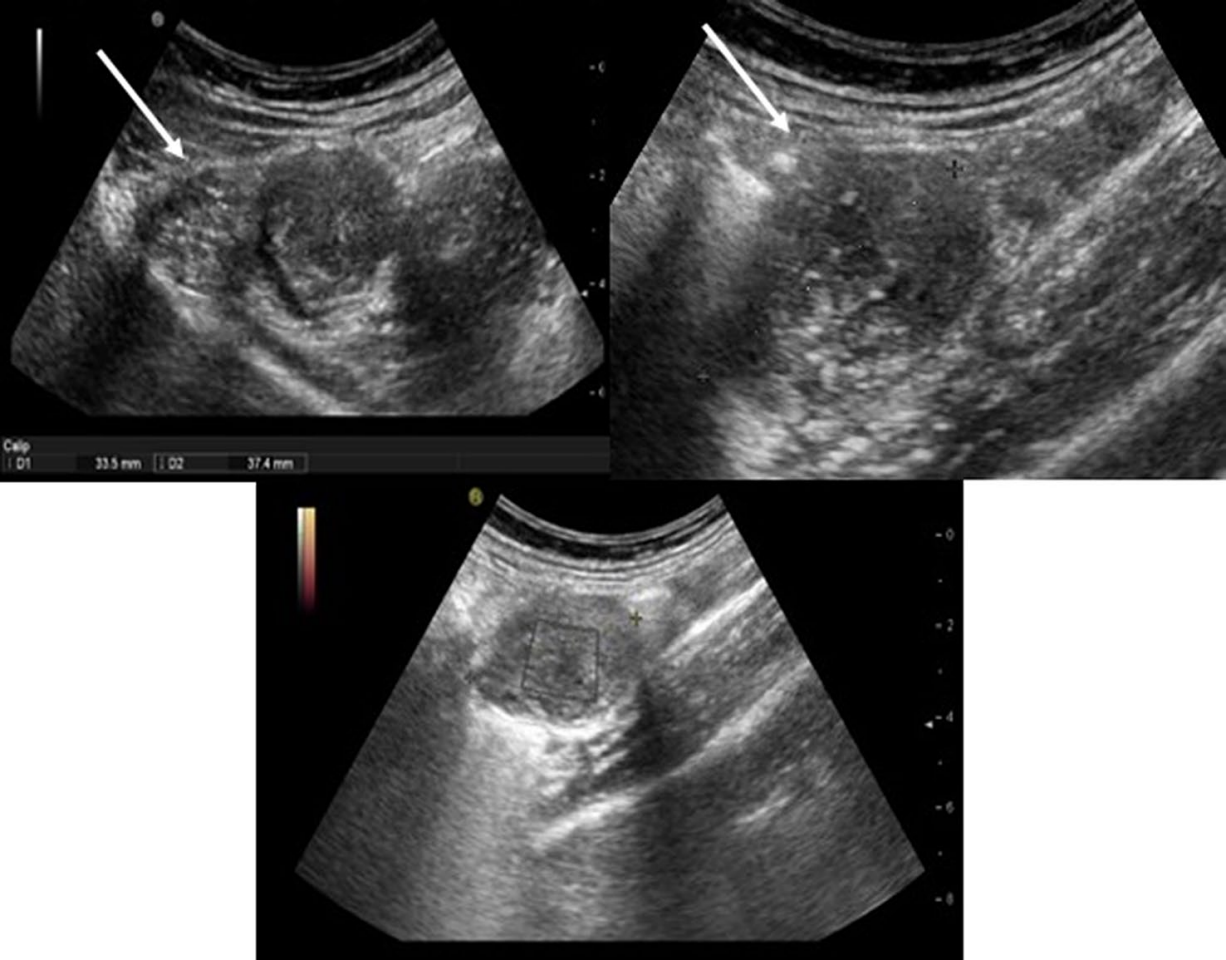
Gastrointestinal neuroendocrine neoplasms (G1). Transabdominal ultrasonography demonstrates a mass involving the appendix as a hypoechogenic lesion with a hyperechogenic border (white arrow)
